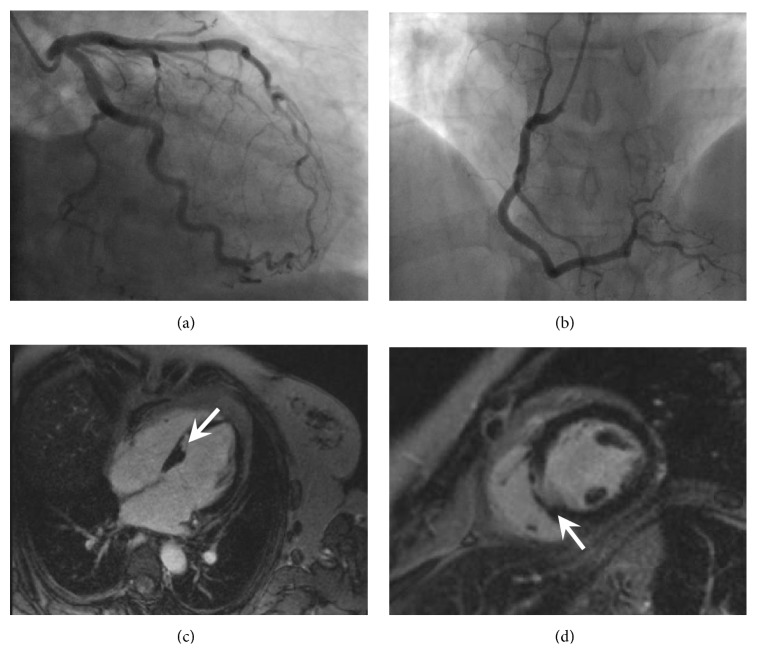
Cardiac imaging: (a) Coronary angiogram showing normal unobstructed left coronary artery. (b) Coronary angiogram showing normal unobstructed right coronary artery. (c) Left ventricle 4 chamber cardiac MRI late gadolinium enhancement image showing area of near transmural infarction in the mid septum. (d) Left ventricle short axis cardiac MRI late gadolinium enhancement image at the papillary muscle level showing area of near transmural infarction of the mid septum.
